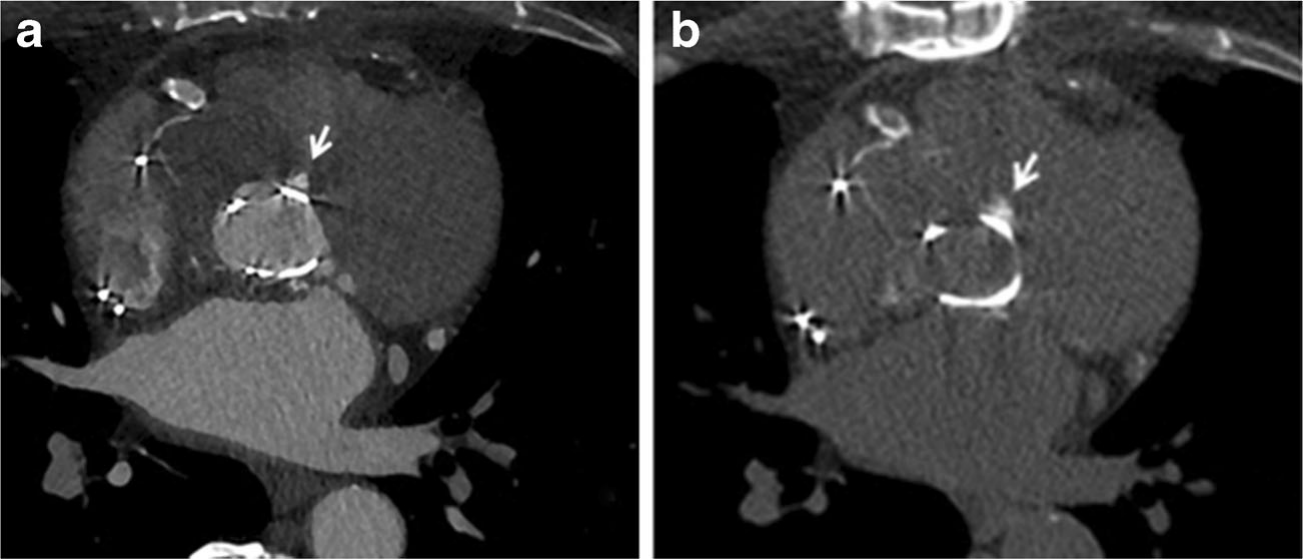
The non-contrast scan can identify sutures with polytetrafluorethylene felt pledgets. These pledgets can be isodense to contrast and mimic paravalvular leakage (PVL) on CT angiography (CTA) (A) and a non-contrast scan (B) can thus be helpful to differentiate between the two. Arrow indicates possible PVL on the CTA and confirms the hyperdense nature of the pledget on the non-contrast scan
